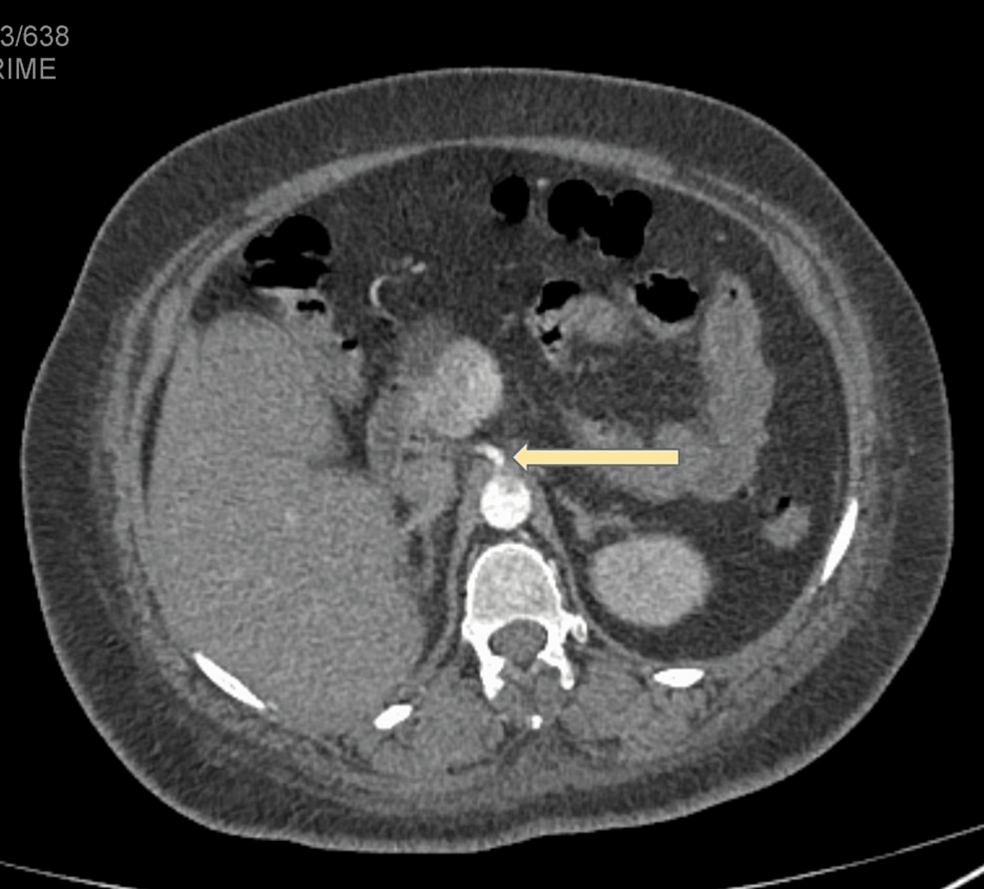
Abdominal CTA image (Axial view) showing the main hepatic artery originated independently from the aorta.
